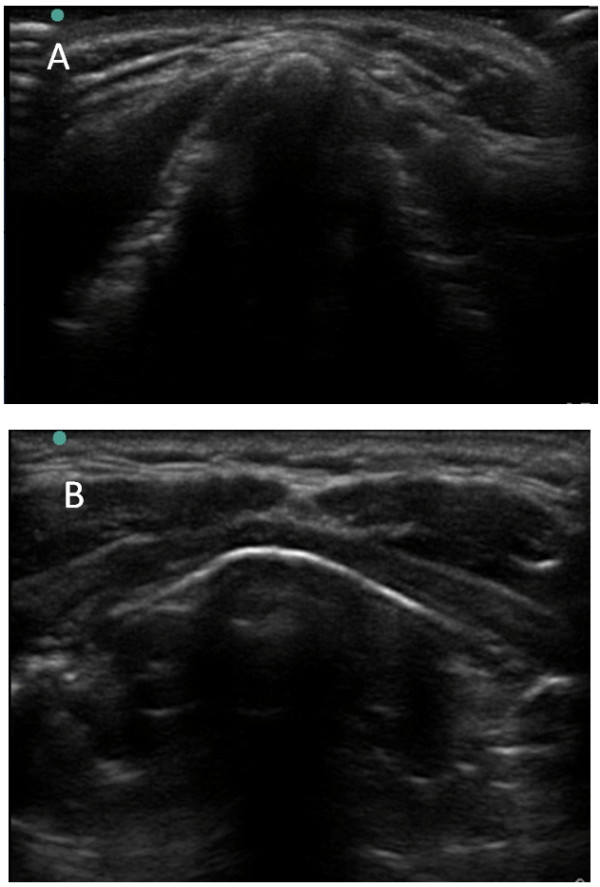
Image of thyroid cartilage using ultrasound in the transverse plane. The subject in panel A is male and is panel B is female.
